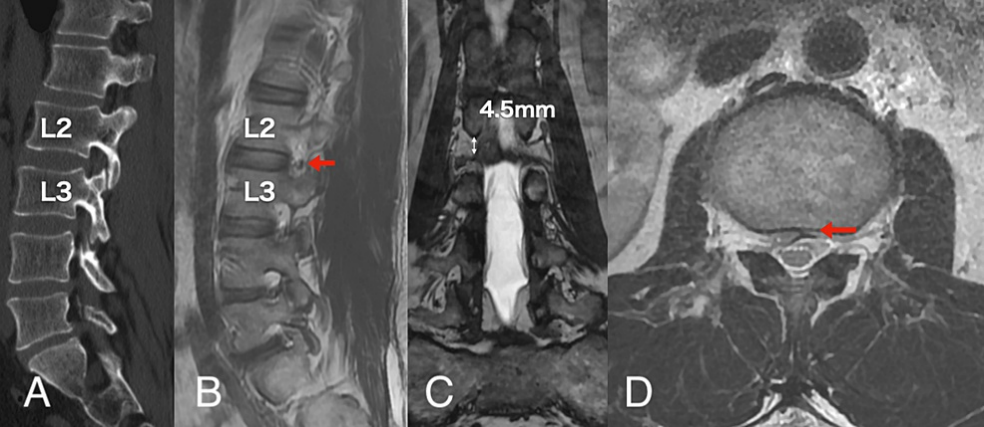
Preoperative imaging (A) CT imaging showed a wide intervertebral foramen at the L23 level. (B) The sagittal MRI showed that the course of the nerve was located at Kambin’s triangle (arrow). (C) The coronal MRI showed that the nerve root was located 4.5 mm distal to the L2 pedicle (arrow). (D) The axial MRI demonstrated that the dura mater was compressed by the herniation that protruded into the spinal canal (arrow).
